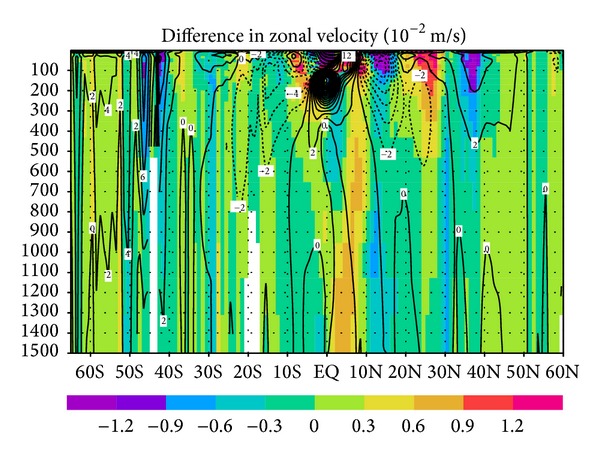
Difference in mean zonal velocity (10^−2^ m s^−1^) during 2001–2007 between GY and CT cases (color) and mean value for the CT case (contours) in a 1500 m vertical cross section along longitude 180°.
